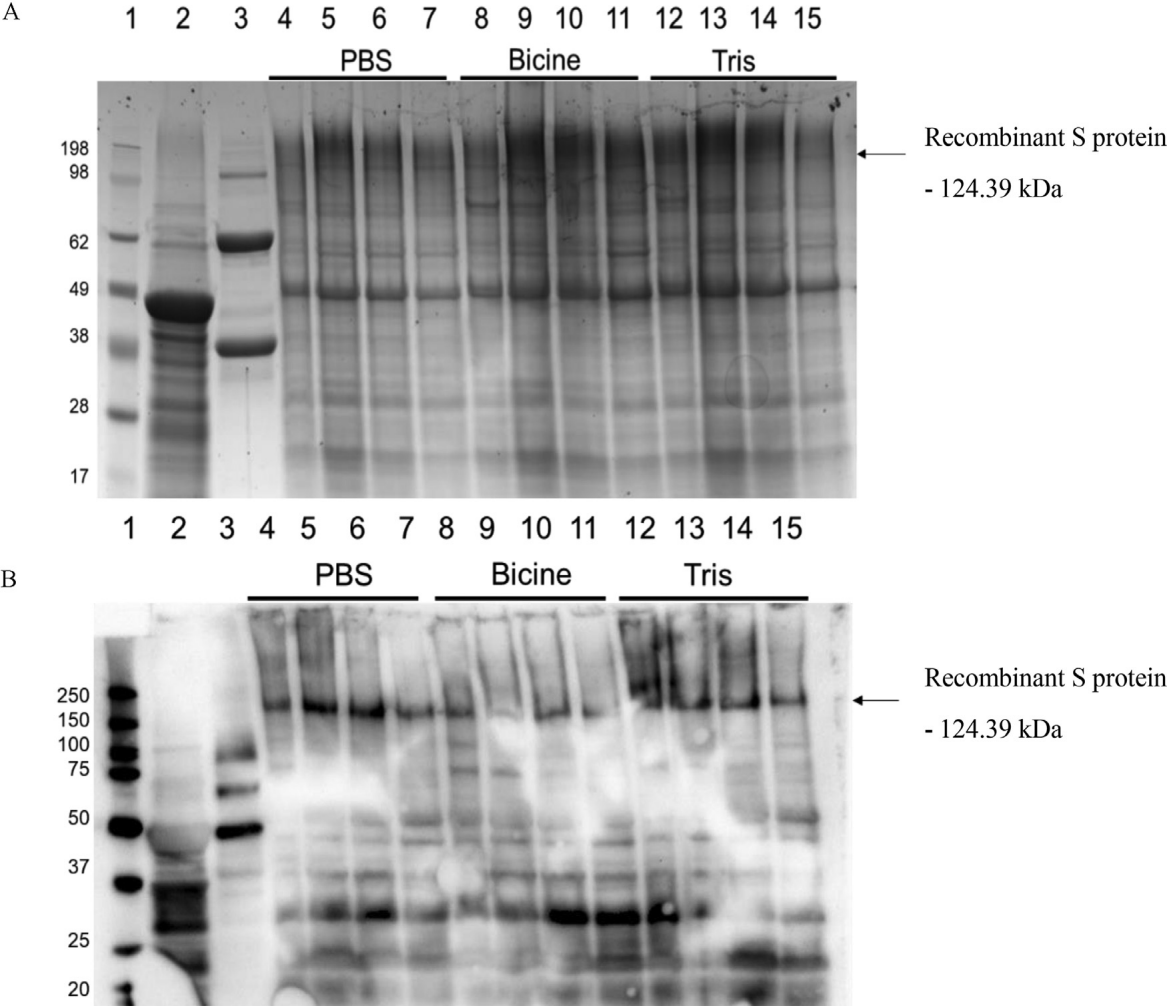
SDS-PAGE (A) and Western blot (B) of plant-produced spike protein (synthetic construct mIBV-S2P-NDV-F^TM/CT^) coinfiltrated at a 4:1 ratio with the NDV matrix protein. Lane 1: molecular weight marker; Lane 2: plant-expressed empty pEAQ-HT vector; Lane 3: purified live QX-like IBV strain ck/ZA/3665/11; Lanes 4 to 7: Fractions 3 to 6 extracted in PBS buffer; Lanes 8 to 11: Fractions 3 to 6 extracted in Bicine buffer; Lanes 12 to 15: Fractions 3 to 6 extracted in Tris buffer.
